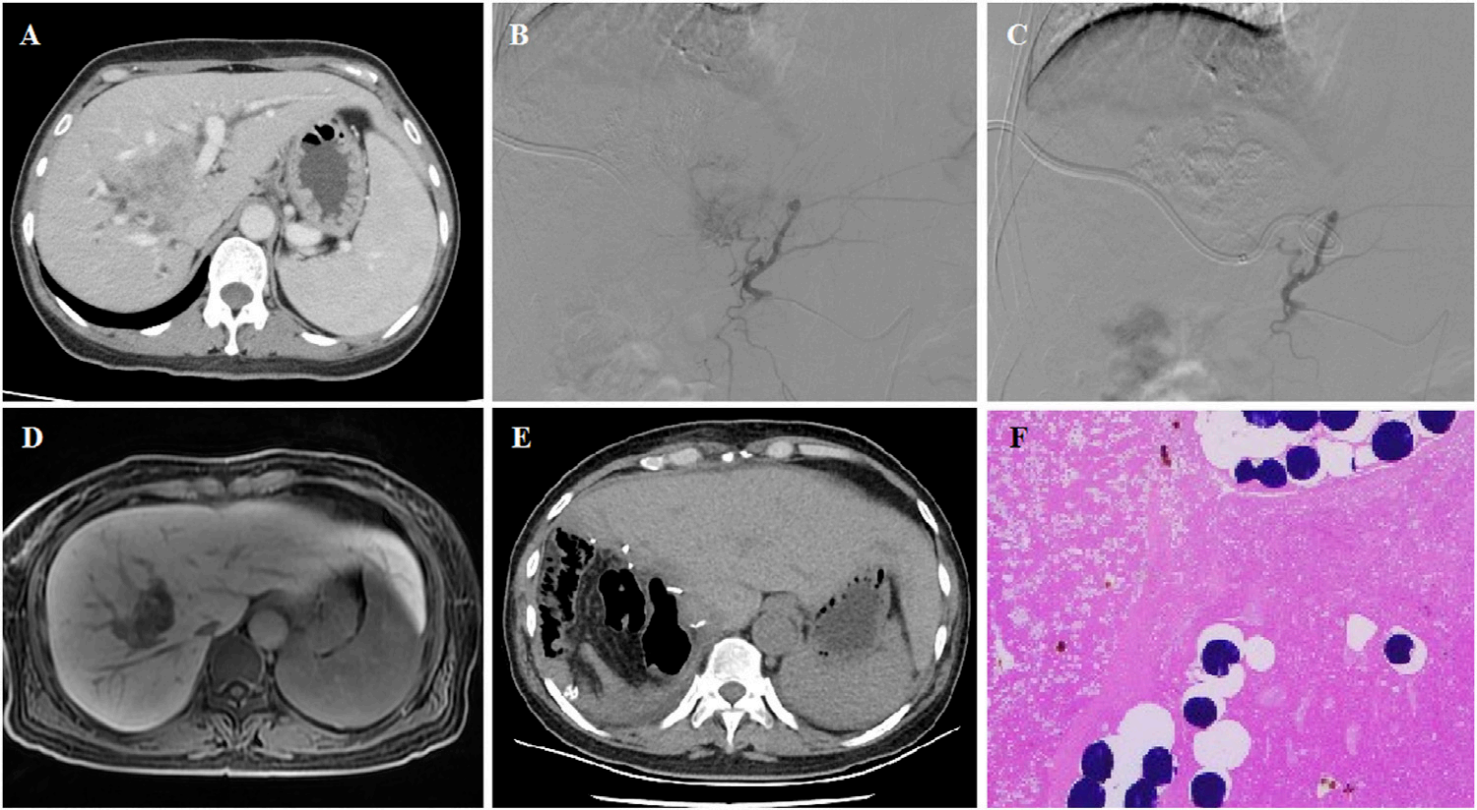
54 years female patient treated with CalliSpheres beads for advanced primary HCC. (A) CT revealed a tumor in the right lobe and the obstructed bile duct. (B–C) Tumor artery was super selectively incubated and embolized by 100–300 μm oxaliplatin-loaded beads. (D) MR revealed a shrunk tumor 4.6 months after DEB-TACE. (E–F) Tumor was resected 4.9 months after DEB-TACE, and no residual tumor was shown by pathological examination.
